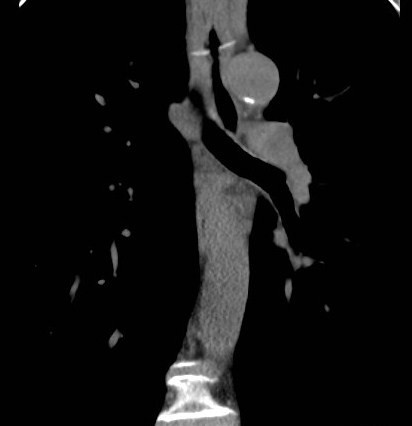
Select coronal image of non-contrast phase CT showing the fish bone lying transversely tenting the oesophagus.
